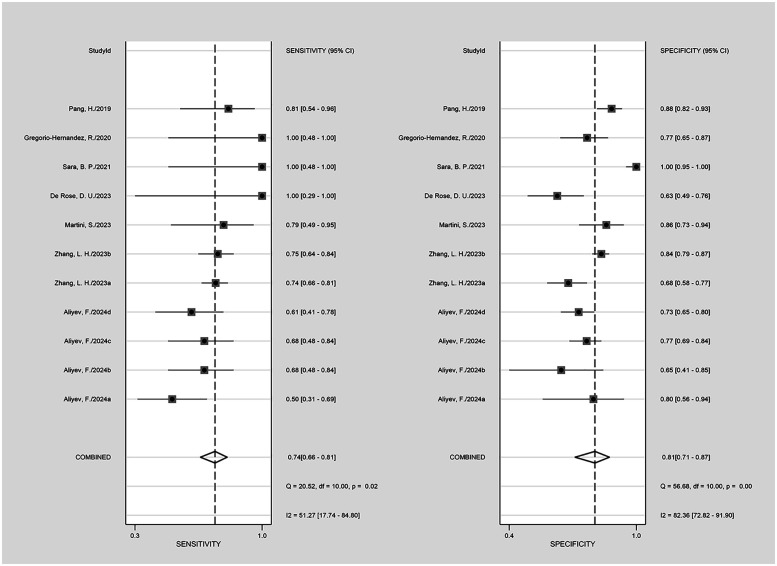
The forest map of the overall sensitivity, specificity, and CI confidence interval of lung ultrasound score for mechanical ventilation needs in the published research.
